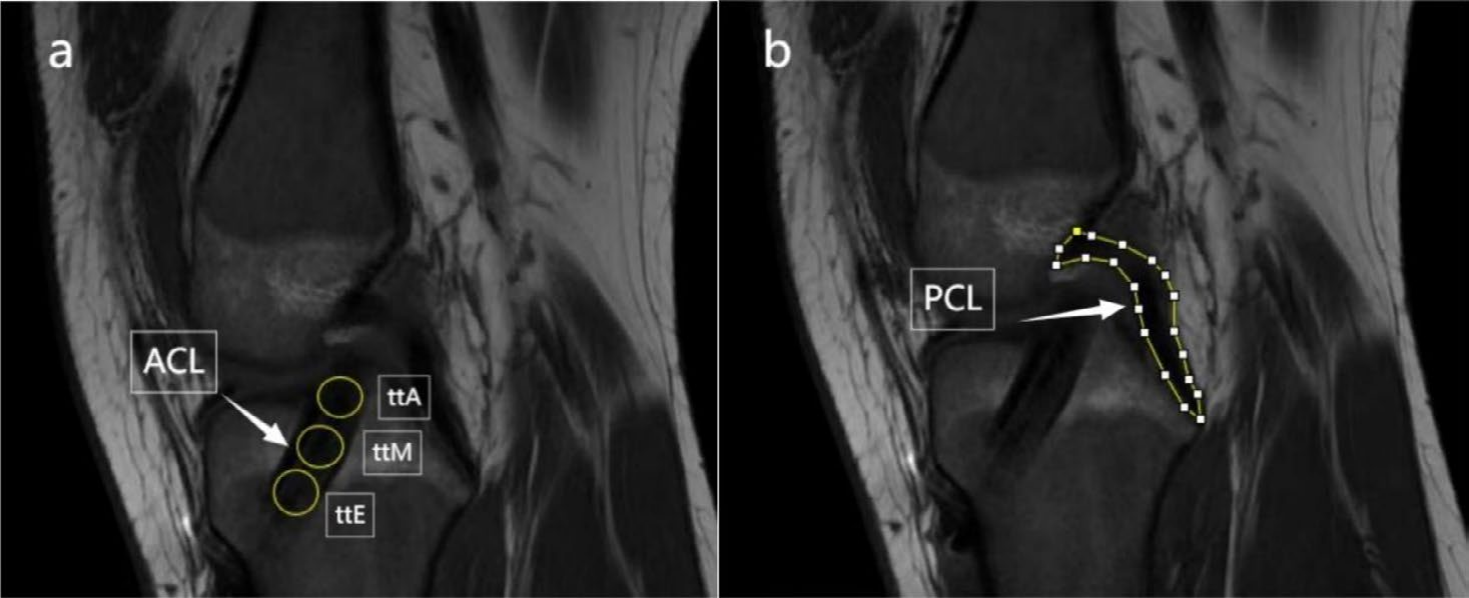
Selecting ROIs on MRI to measure the signal intensity of the ACL graft (a) and the PCL (b). ACL, anterior cruciate ligament; ttA, tibial tunnel aperture; ttE, tibial tunnel exit; ttM, tibial tunnel midsection; PCL, posterior cruciate ligament; ROI, region of interest.
